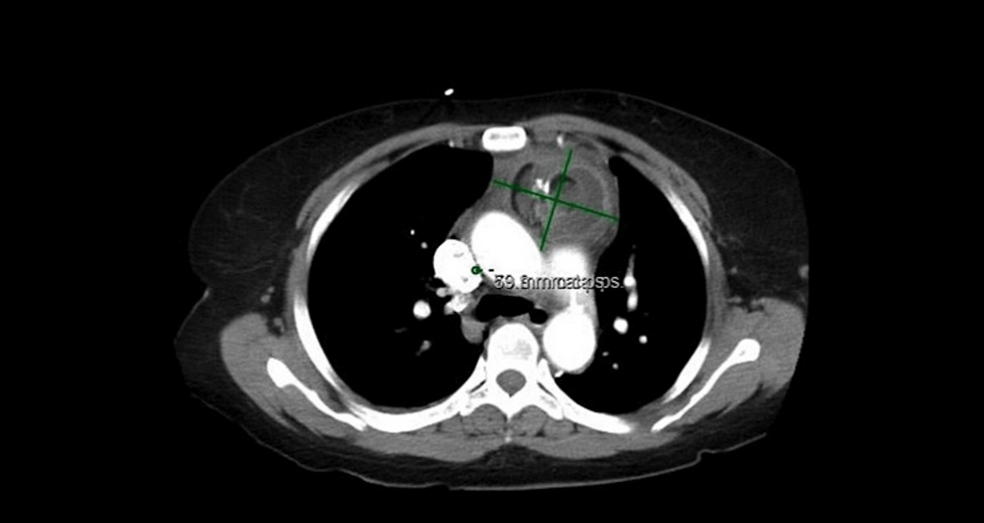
CT angiography of the chest revealing a mediastinal mass containing soft tissue, fat, fluid, and areas of calcification. CT: Computed tomography
